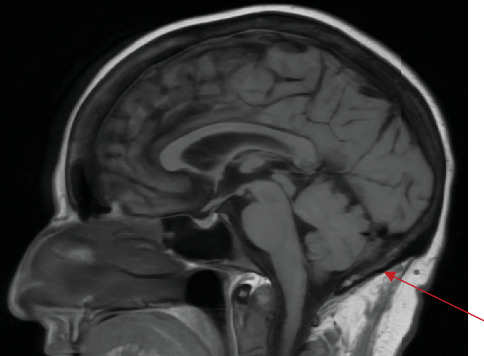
Sagittal MRI showing posterior cerebellar leptomeningeal enhancement.
